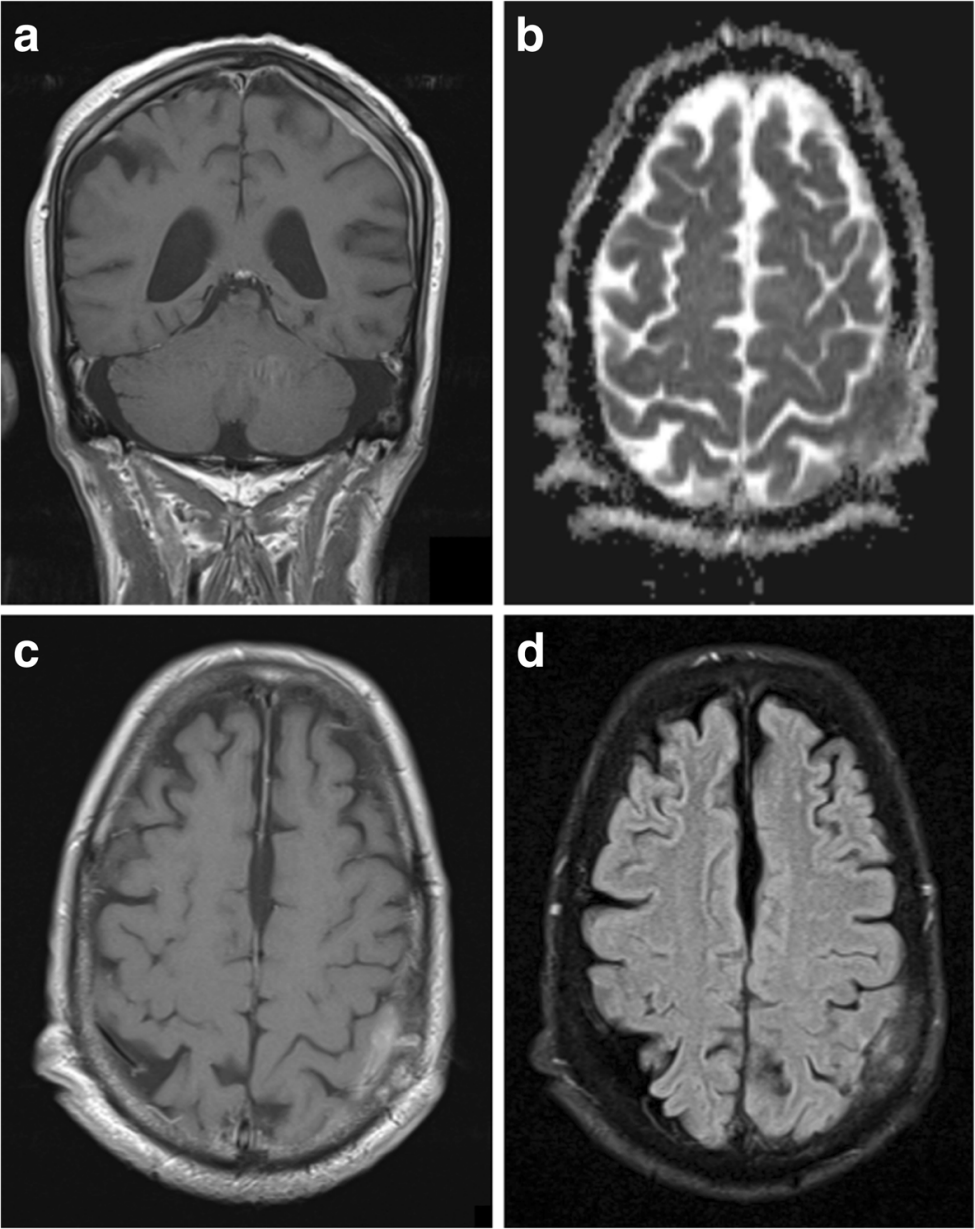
IgG4-related hypertrophic pachymeningitis with skull invasion. a Post-contrast coronal T1-weighted MR image showing linear dural thickening and enhancement overlying the left cerebral convexity. Note that the overlying calvarial bone marrow signal is abnormal. b Apparent diffusion coefficient (ADC) map showing restricted diffusion in the lesion overlying the left parietal region. c Post-contrast axial T1-weighted image of the same lesion with enhancing soft tissue seen invading the skull. d Axial fluid attenuated inversion recovery (FLAIR) image showing the lesion is predominantly hypointense due to fibrosis with foci of hyperintensity
